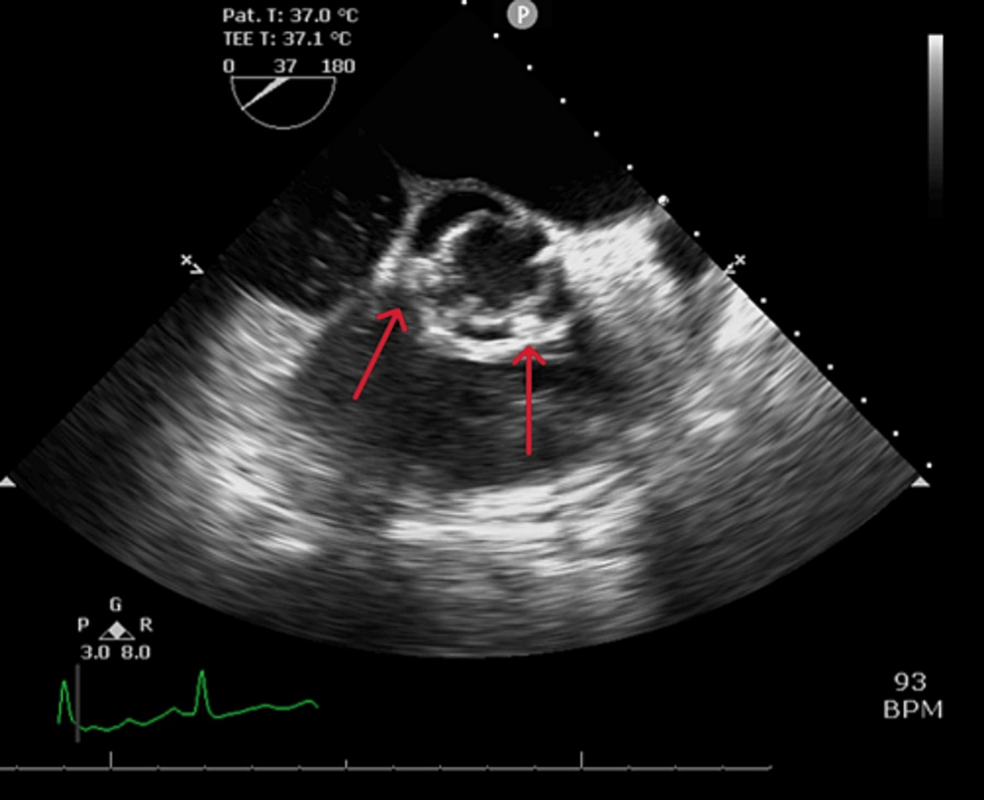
BAV vegetations on transesophageal echocardiogram BAV: bicuspid aortic valve
